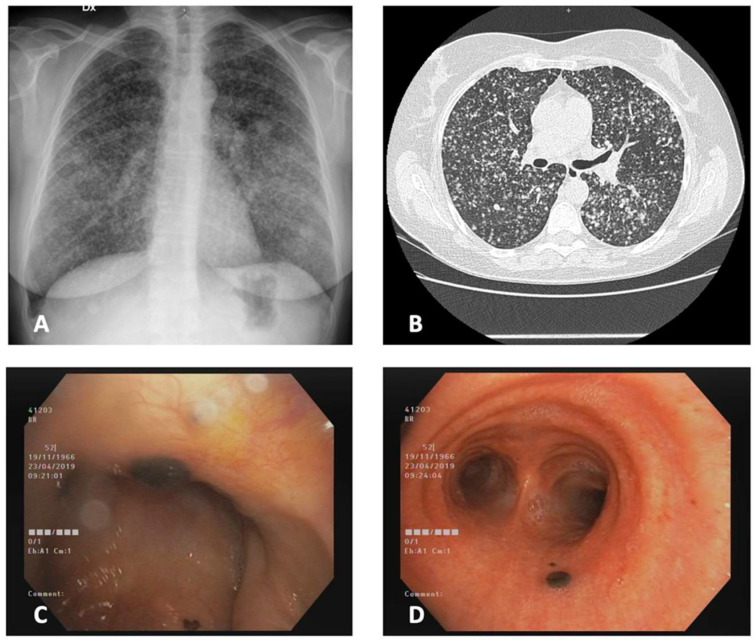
Case 3. (A) Antero-posterior chest X-ray at presentation showing a diffuse reticulonodular alteration. (B) CT scan at presentation, showing multiple micronodules with a random distribution, suggestive of metastasis. (C,D) nasal and tracheobronchial black plaques, consistent with metastasis. CT, computerized tomography.
